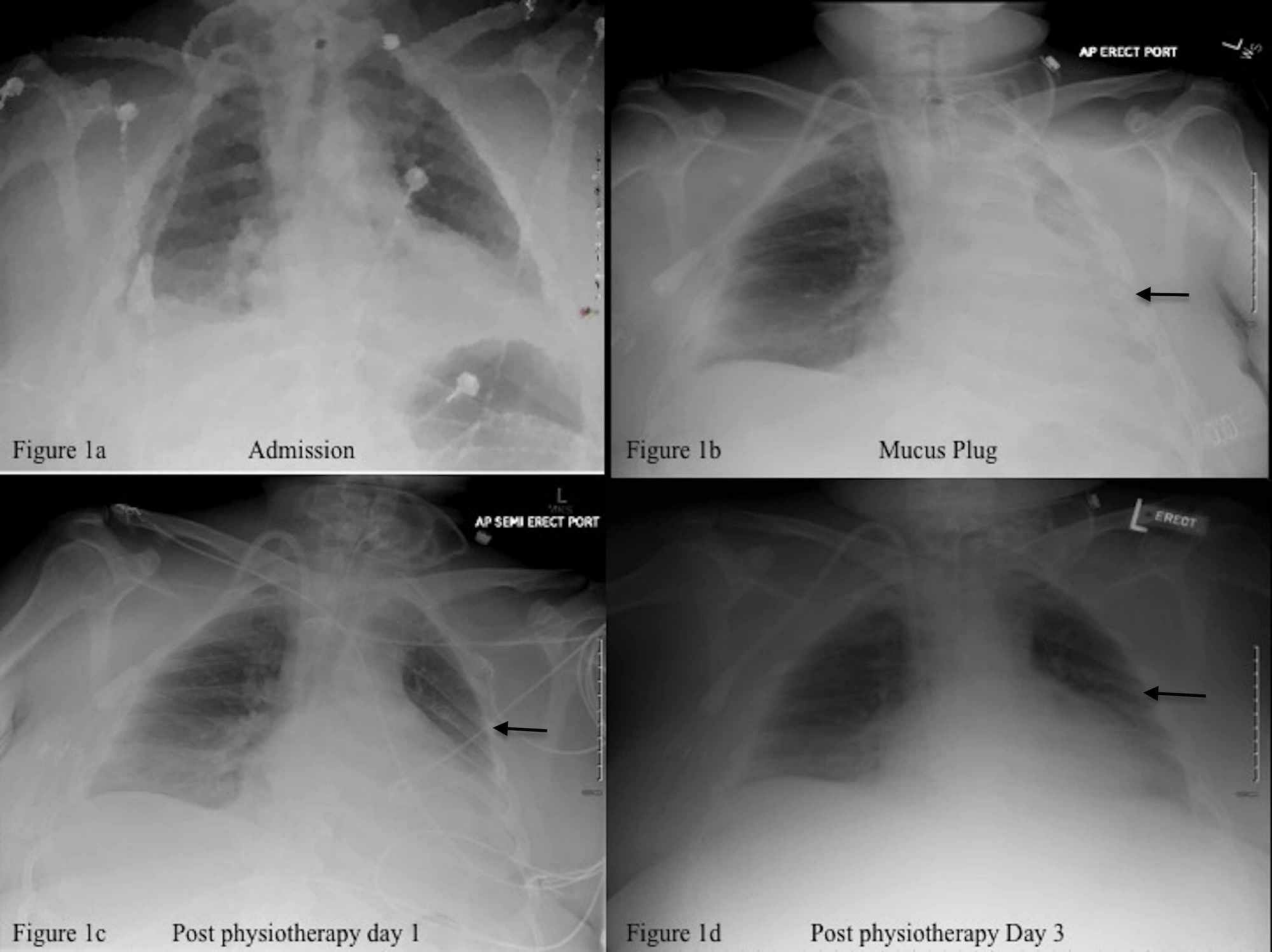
Chest X-rays of the patient's progress before and after intervention 1a) Tracheostomy tube in position of trachea, dialysis catheter in the upper right atrium, cardiomegaly, pulmonary congestion, bilateral pleural effusions, and left lower lobe infiltrates. 1b) Minimal aeration of the left lung, volume loss, and partial atelectasis (arrow). 1c) Improved aeration of left lung post respiratory physiotherapy (arrow). 1d) Normal aeration of the left lung (arrow).
